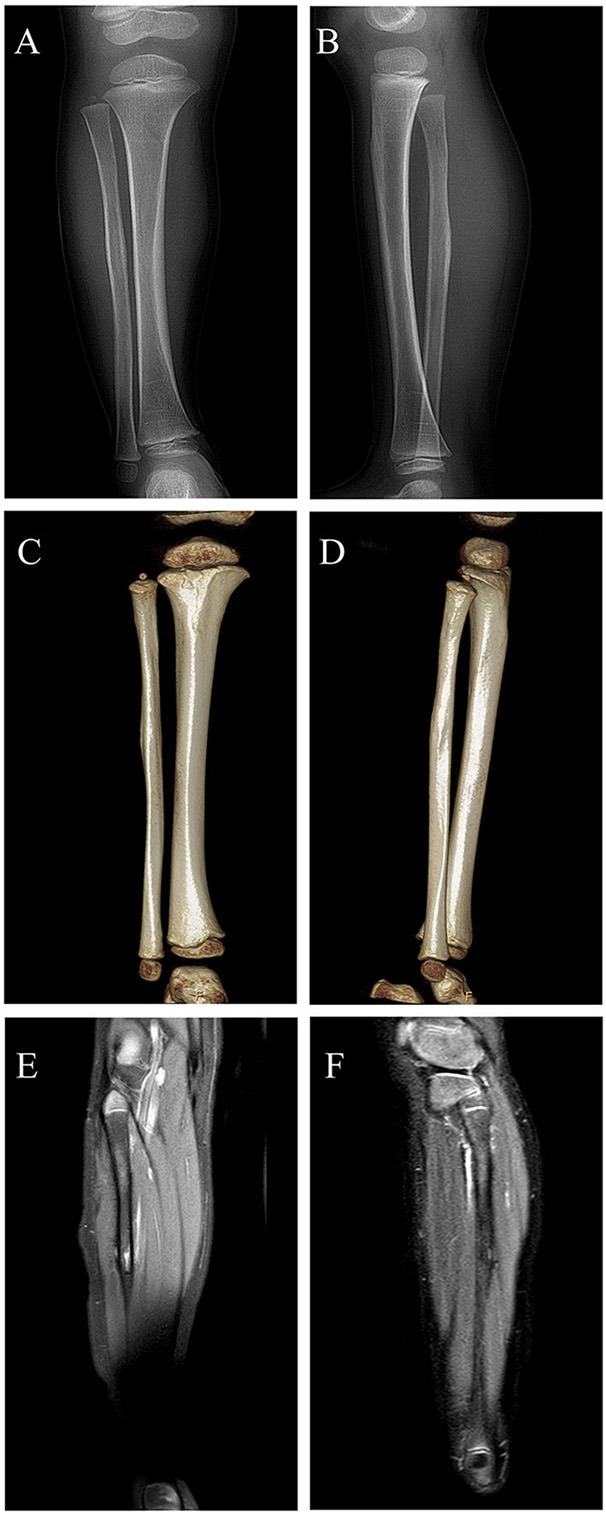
Digital radiograph at 2 years postoperatively: (A,B) Radiographs showing reformation of the cortex of the proximal fibula; (C,D) 3d CT reconstruction demonstrating both uniform bone mineral density and continuous cortical of right proximal fibula; (E,F) MRI revealing remarkable regression of lesion without evidence of local recurrence.
